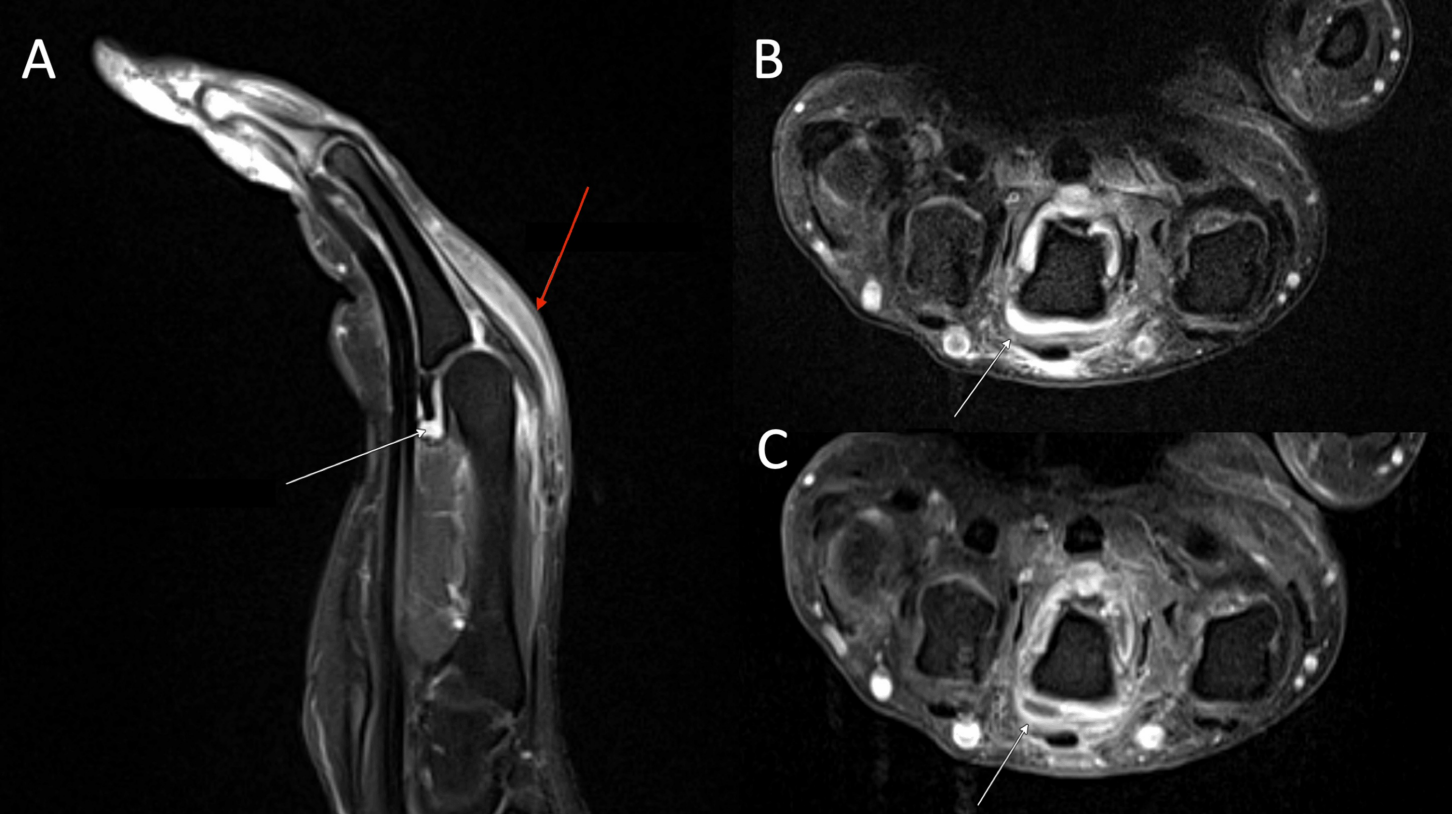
MRI of the right hand showing T1-weighted sagittal (A), proton density with contrast (B), and T1-weighted post-contrast (C) views showing synovial enhancement, effusion, and periarticular edema surrounding the third right MCP joint. (A) Joint capsule hyperenhancement is indicated by the white arrow, and subcutaneous hyperenhancement is indicated by the red arrow. (B) Synovial hyperenhancement is highlighted by the white arrow. (C) Effusion is shown by the white arrow. MRI: magnetic resonance imaging; MCP: metacarpophalangeal
